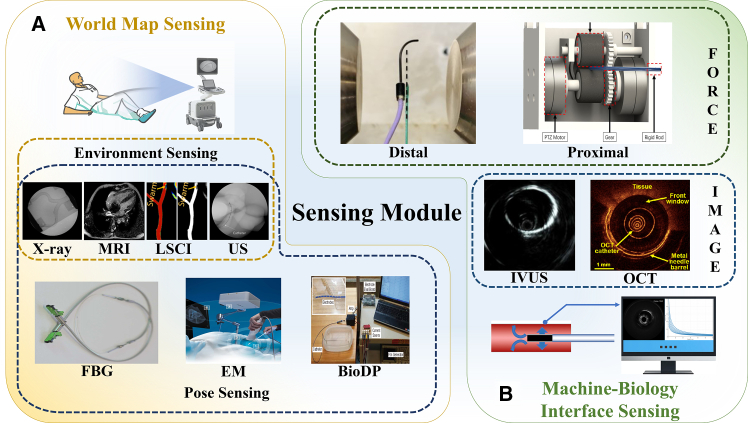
Classification of various sensors used in EIRSs Sensing modalities are grouped by function: (A) World map sensing supplies global information, which is categorized as environment sensing (X-ray,^71^ MRI,^72^ LSCI,^73^ and US^74^) and pose sensing (FBG, EM, and BioDP^75^). (B) Machine-Biology Interface Sensing captures local interaction, which is subdivided into force-based (distal sensor,^40^ proximal sensor^74^) and image-based (IVUS^76^ and OCT^77^). Abbreviations: MRI, magnetic resonance imaging; LSCI, laser speckle contrast imaging; US, ultrasound; FBG, fiber Bragg grating; EM, electromagnetic; BioDP, bio-dielectric property; IVUS, intravascular ultrasound; OCT, optical coherence tomography.
